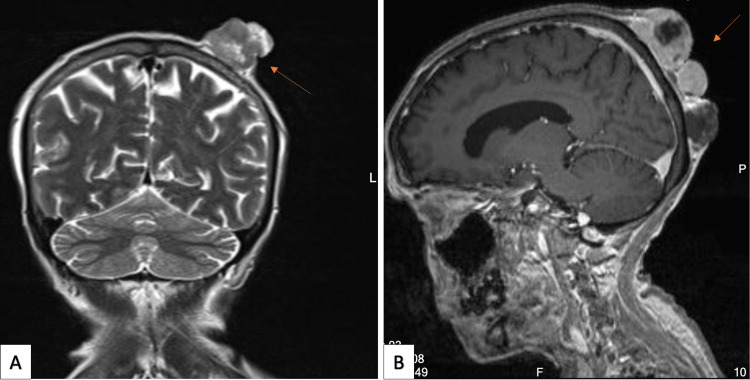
MRI of the brain with progression of the scalp metastatic lesions. (A) Coronal view of the brain. (B) Sagittal view of the brain.
